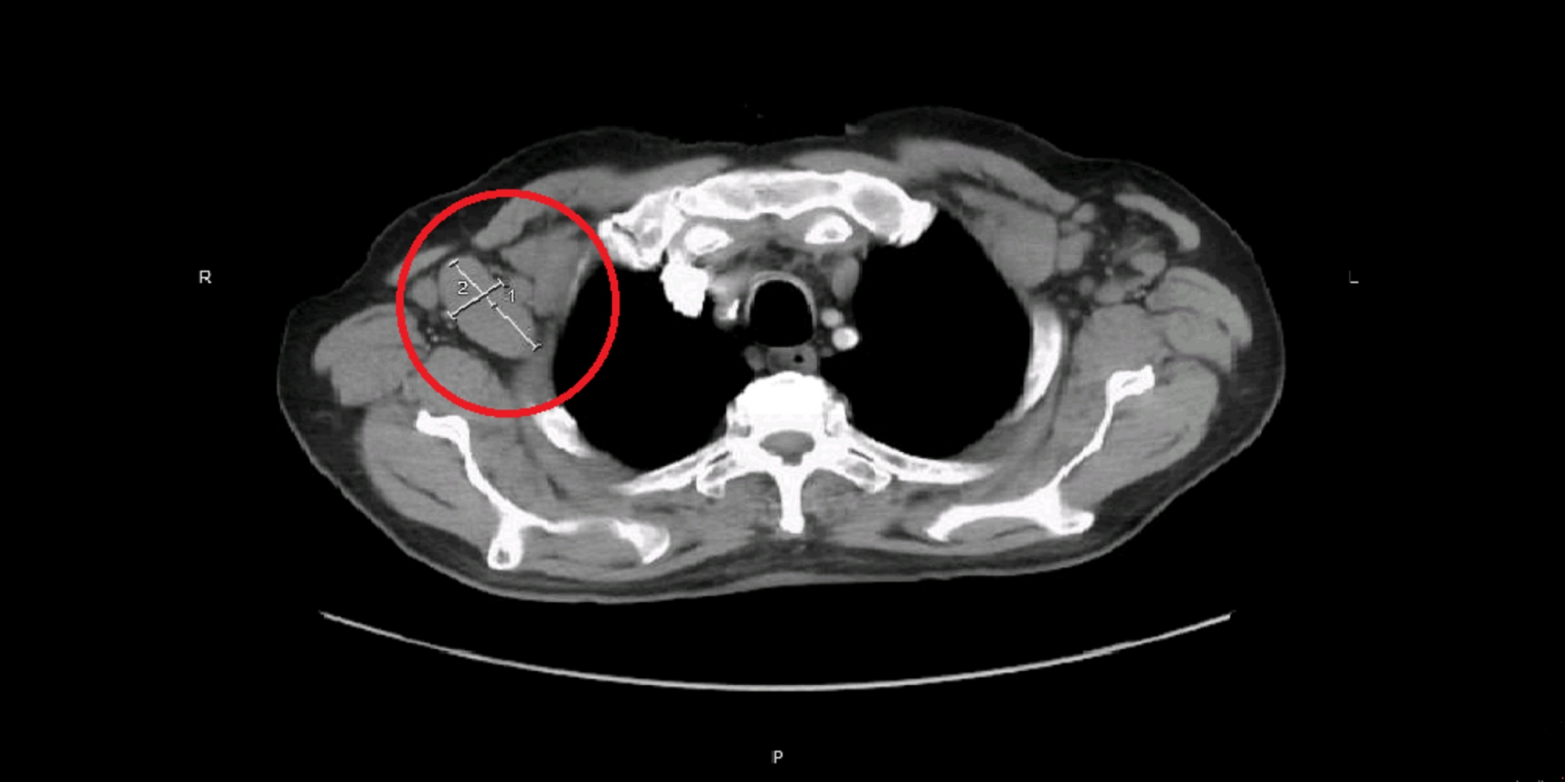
CT scan chest. Axillary lymphadenopathy (red circles).
